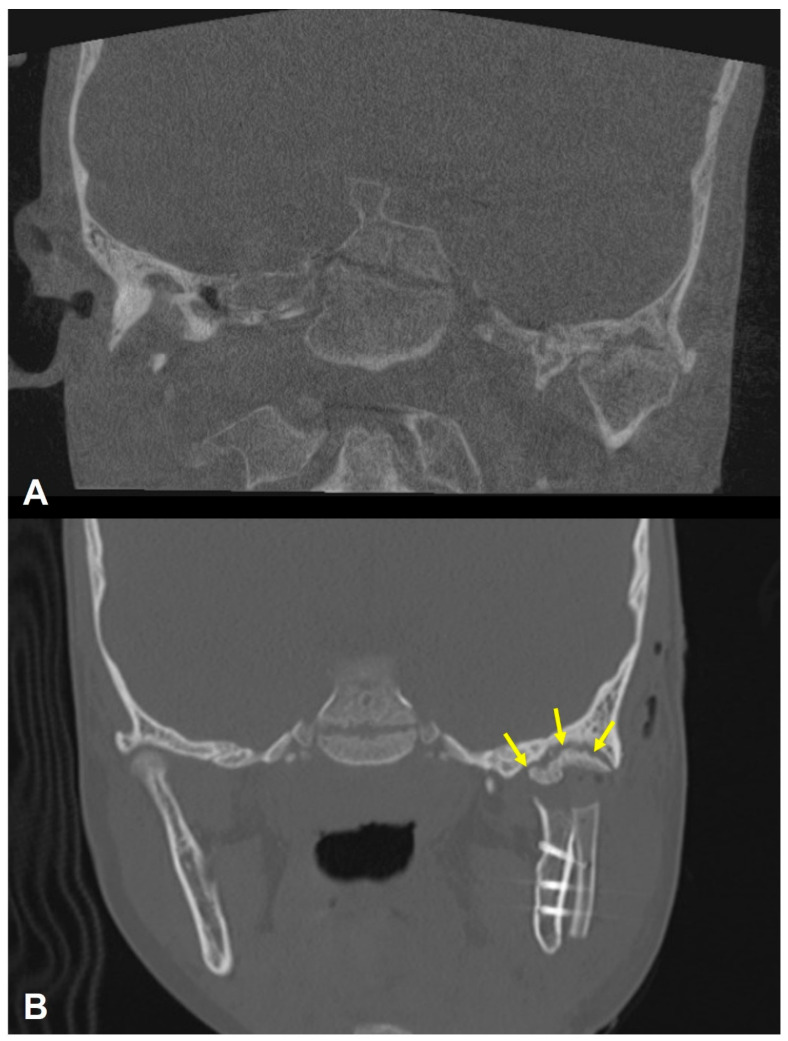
(A) Preoperative coronal CBCT demonstrating fibro-osseous fusion of the left condyle with the skull base. (B) Postoperative coronal CT demonstrating a partially removed ankylotic mass (yellow arrows).
